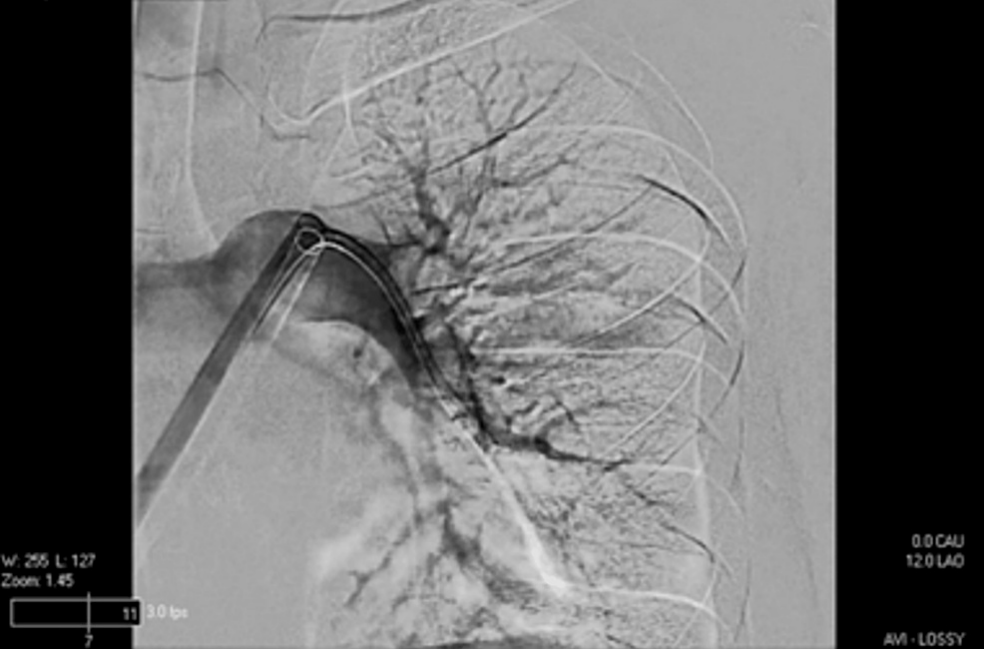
Ultrasound-guided right femoral vein access catheterization of the left lung
